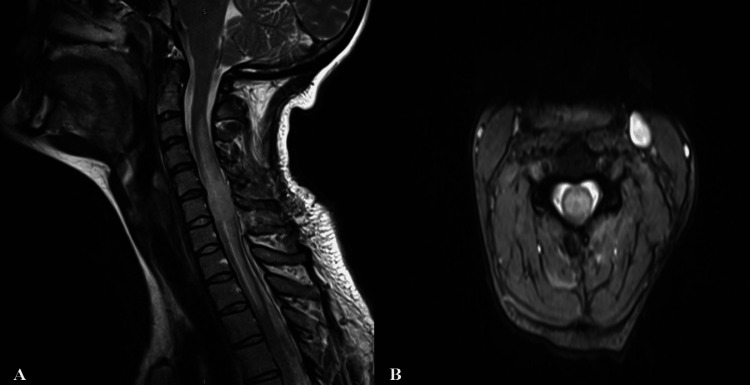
Cervical sagittal (A) and axial (B) MR images of the patient on the seventh postoperative day demonstrating diffuse myelomalacia
